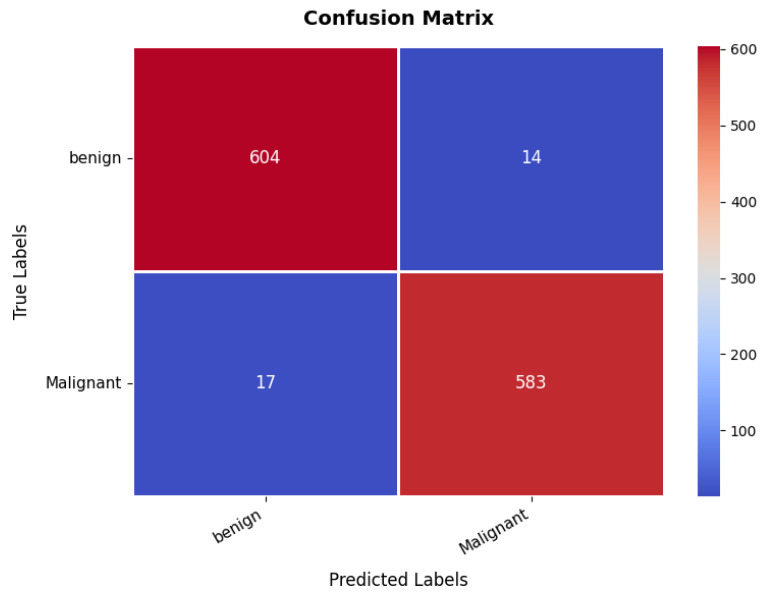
Confusion matrix of breast ultrasound dataset.
